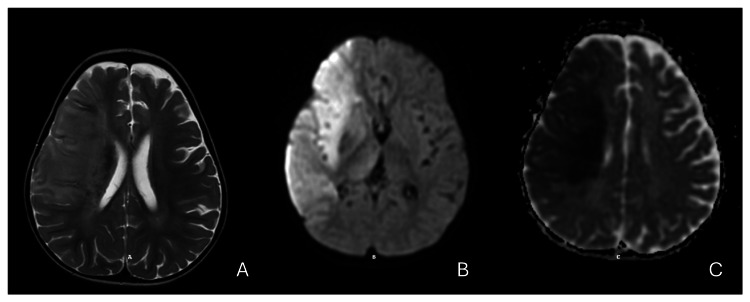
A) T2-weighted imaging: it shows a hyperintense signal seen in the deep white matter following the distribution of the right middle cerebral artery with brain edema and midline shift. B) DWI (diffusion-weighted imaging): showing a bright hyperintensity (restricted diffusion) noted in the right deep white matter area, and C) ADC (apparent diffusion coefficient) map showed a corresponding low signal (hypointensity) in the same region, confirming true restricted diffusion. This confirms acute ischemic infarction involving the right-sided temporoparietal peritoneal middle cerebral artery.
